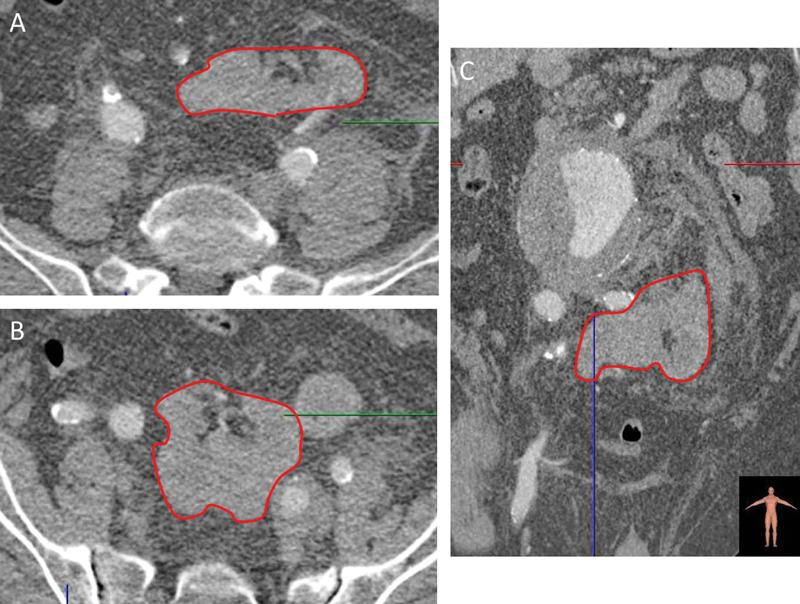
Computed tomography angiogram imaging of congenital fused pelvic kidney or pancake kidney. (
